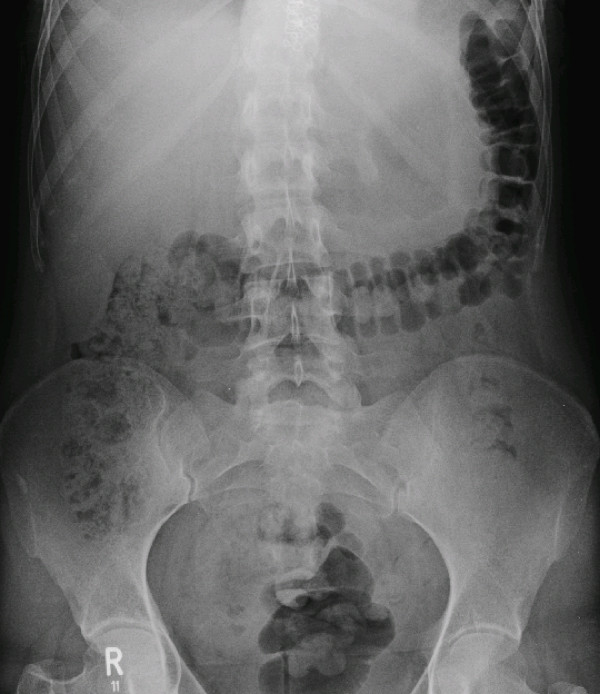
Radiograph after therapy.
